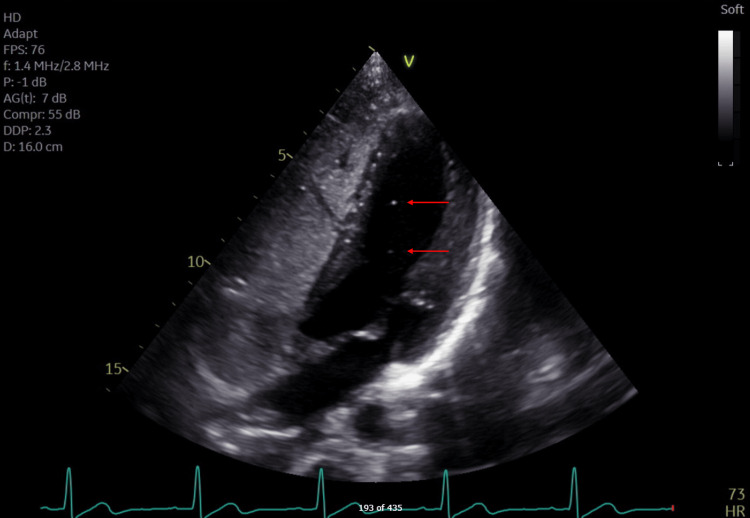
Transthoracic echocardiogram of the patient undergoing an agitated saline study. Complete opacification is noted in the right atrium and ventricle, signifying an adequate study, while two bubbles are noted with red arrows, signifying a Grade I positive agitated saline study.
